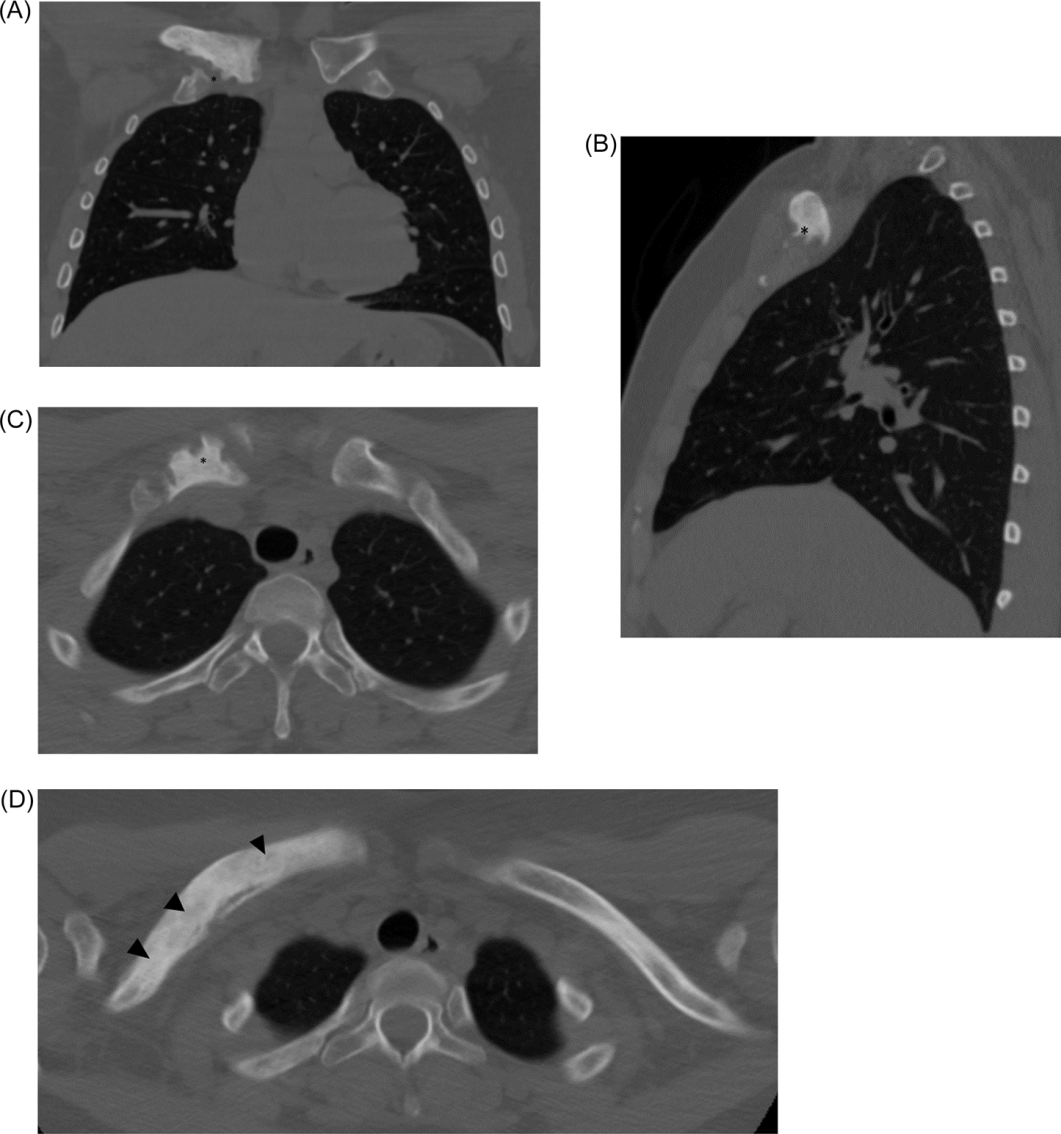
Chest CT without contrast obtained, September 2018, revealed a diffuse and expanded right clavicle with cortical breakthrough with scalloping and erosions. Coronal (A) and Sagittal (B) views depicting hook-like osteophytes in the inferior-medial clavicle (*). Axial (C, D) views demonstrating bony erosion (*) and marrow canal obliteration (black arrowheads).
